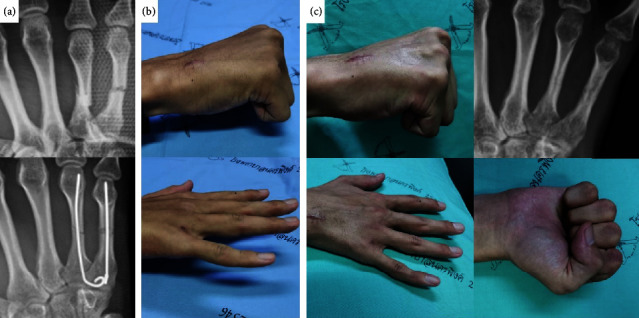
A 23-year-old male with fracture of the fourth and fifth metacarpal shafts: (a) pre and postoperative radiographs of his right hand, (b) clinical pictures of the patient's hand ROM at 6 weeks postoperative, (c) clinical pictures of his ROM at 3 months after surgery. The radiograph showed bone union. He regained full ROM. His DASH score was 5 and his grip strength was 77.46% compared to his left hand.
